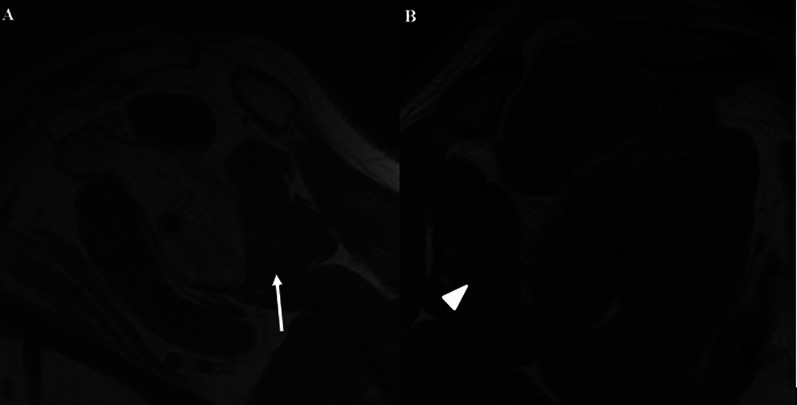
MRI sagittal cuts of the shoulder for two patients who underwent the remplissage procedure: A the* white arrow* reveals mild fatty infiltration; B the* arrowhead* shows the absence of fatty streaks inside the belly of the infraspinatus muscle
